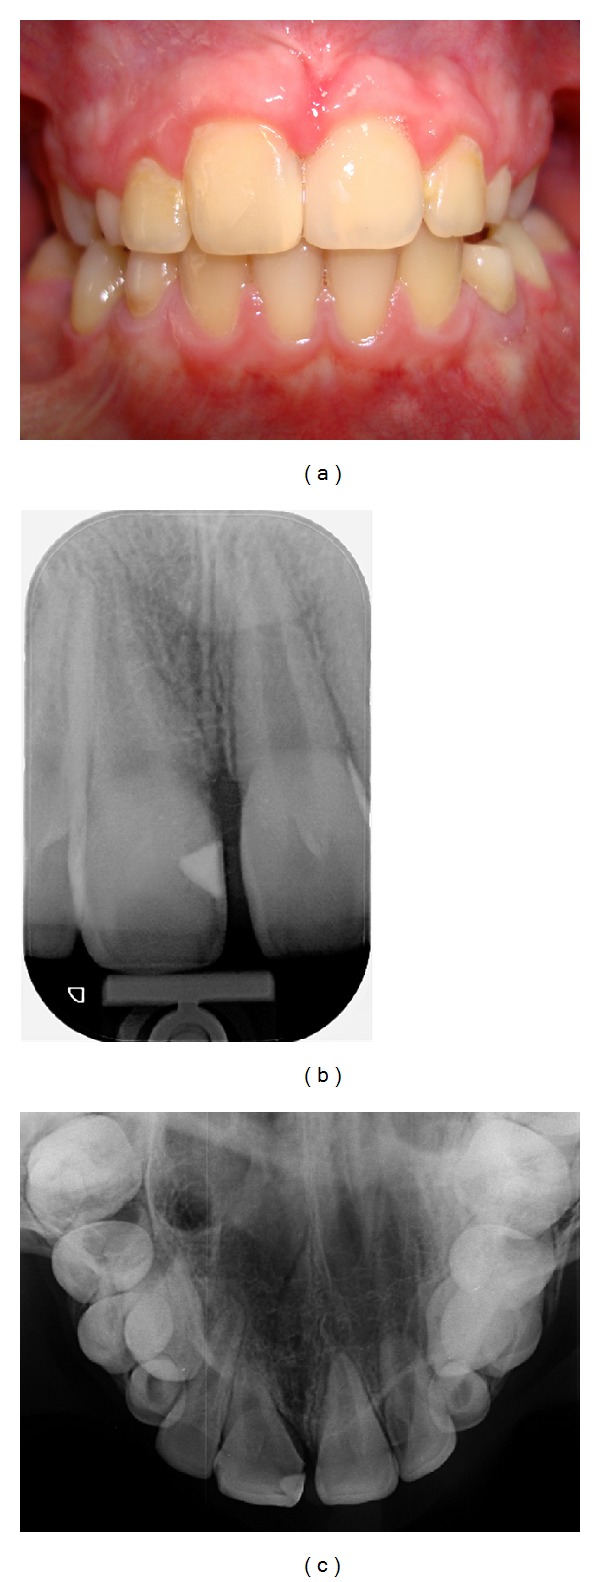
Clinical and radiographic appearance at 18-month followup.
